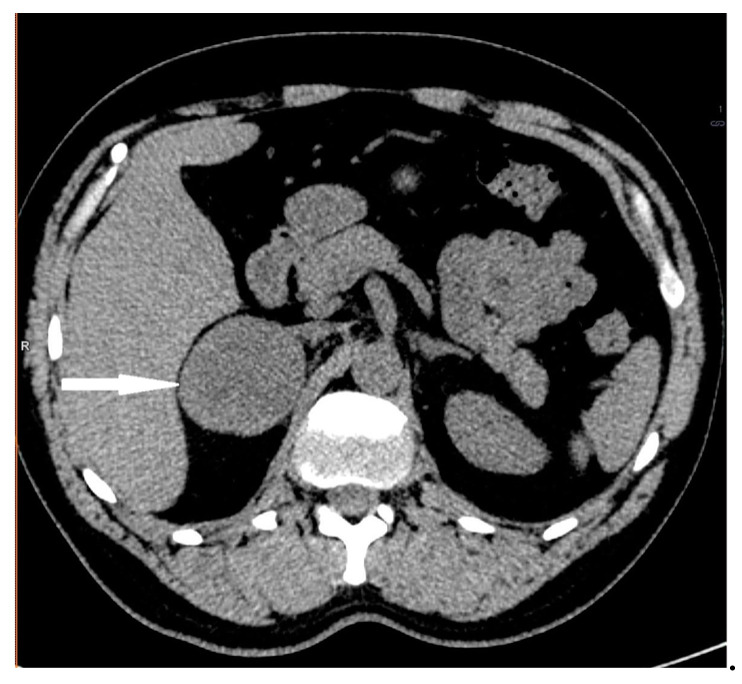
Pheochromocytoma. Abdomen CT, transverse cross-section.
